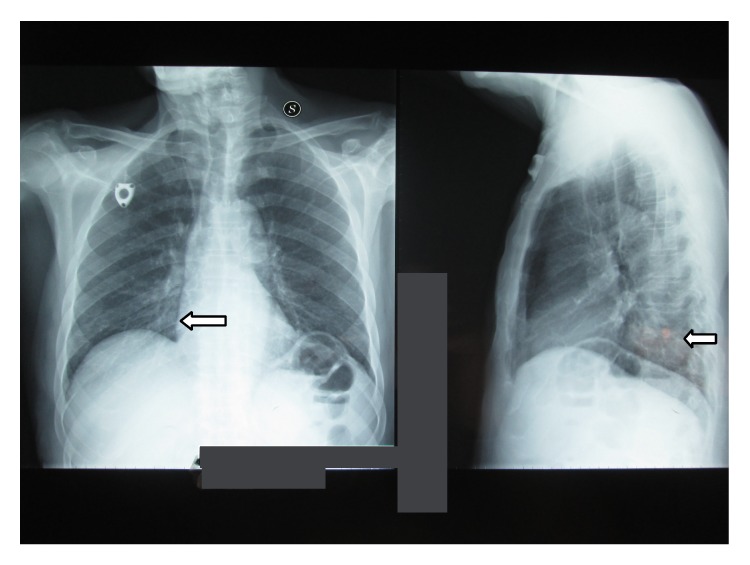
Chest X-ray at the admission.
